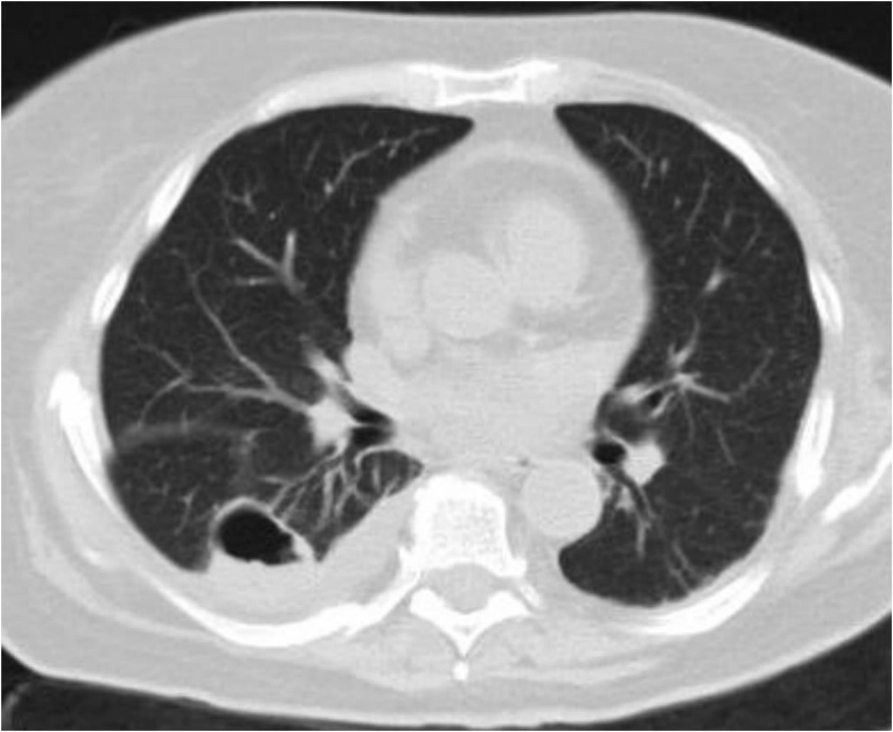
A computed tomography evaluation about one month after admission showed complete resolution of the air-filled cavitary lesion anteriorly. In the area of the contusion, a large thin-walled air cavity displaying an air-fluid level is evident.
